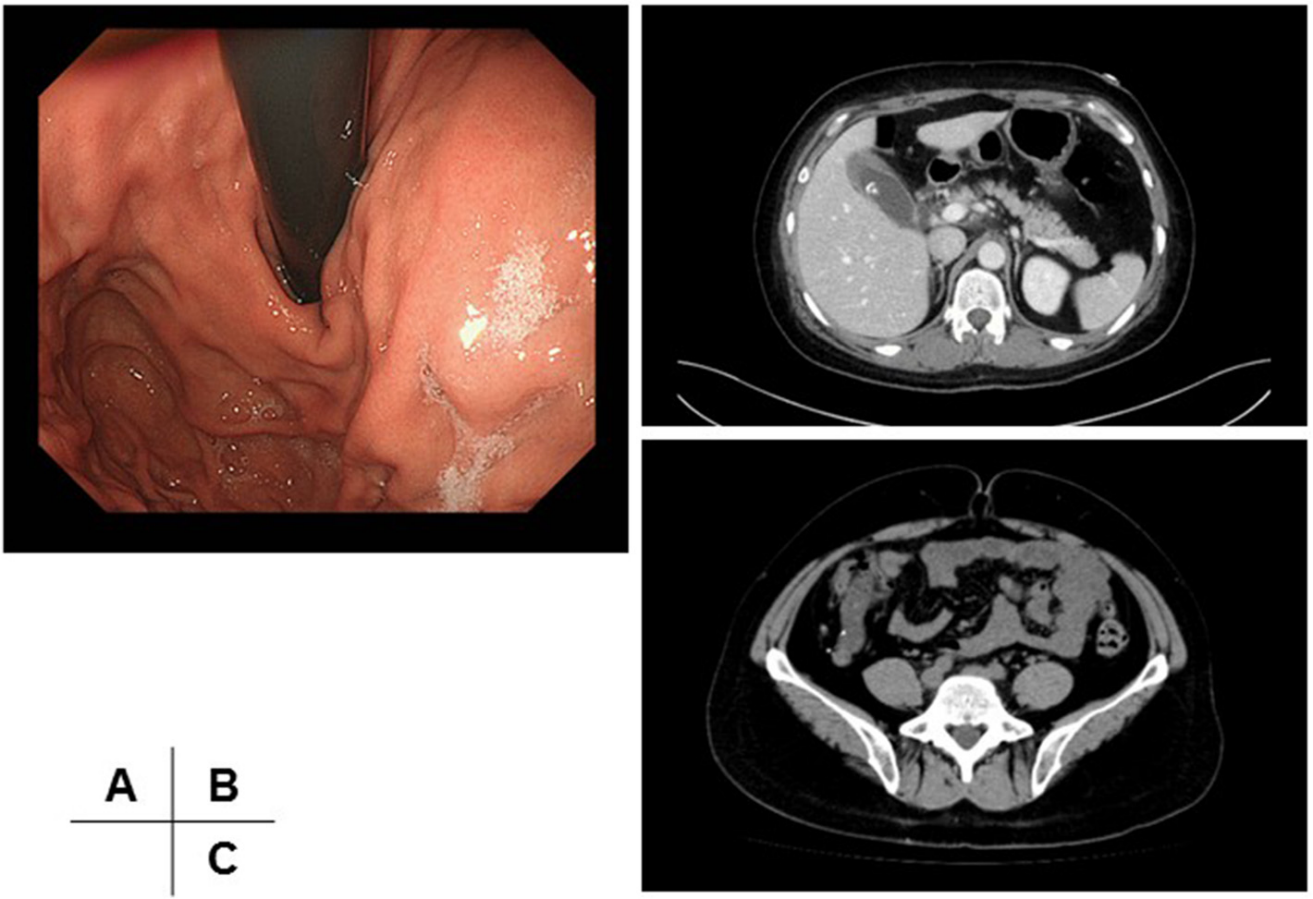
Images of Case 2. a Upper gastrointestinal endoscopy showed a slight hiatal hernia. b CT revealed cholelithiasis. c Abdominal CT identified a small umbilical hernia
